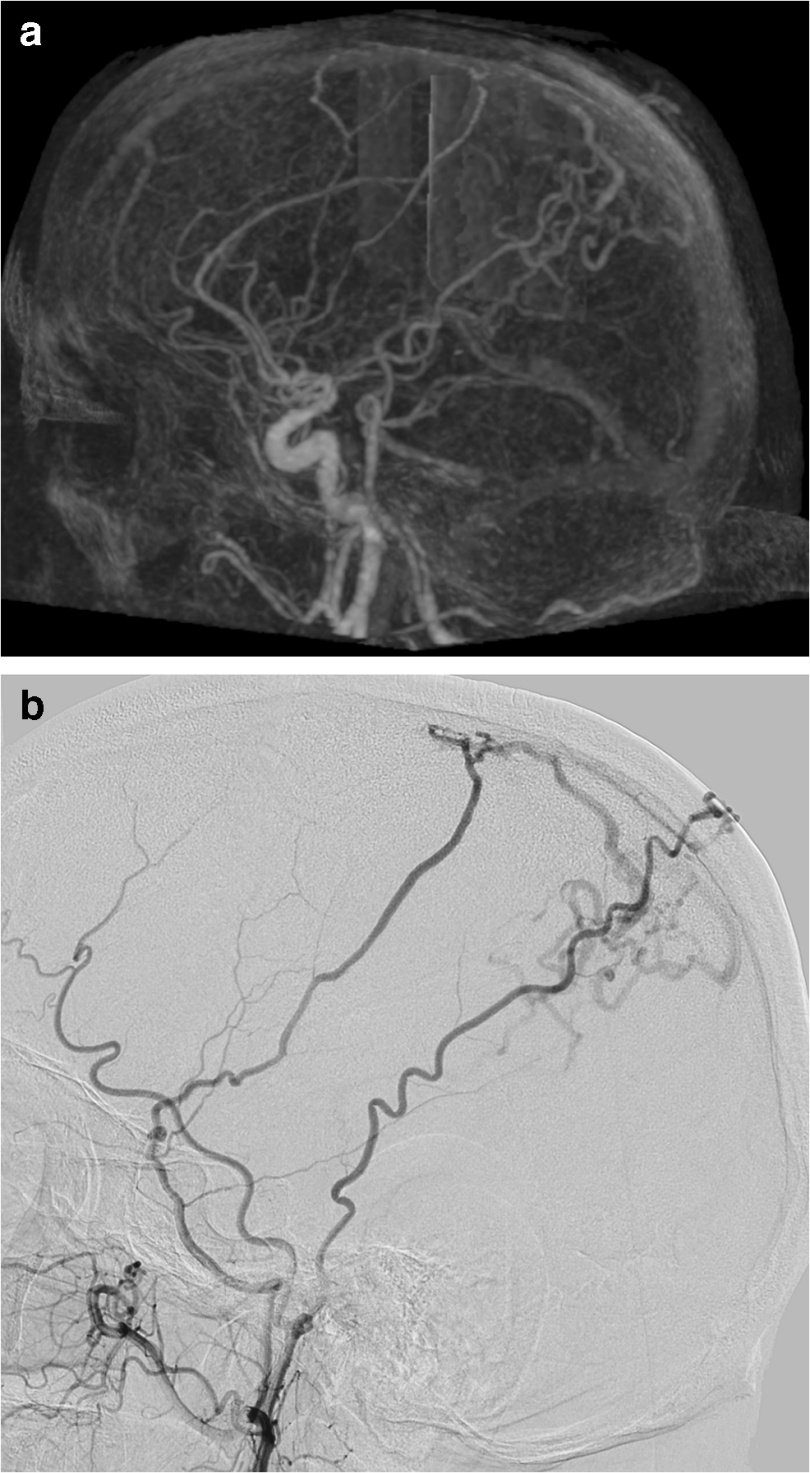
Panels a and b (right external carotid injection) are from the case with 4D-CTA misclassification. The early draining veins are clearly visible in both studies. They were thought to represent drainage from a bAVM with the 4D-CTA while DSA clearly demonstrates a dAVF
